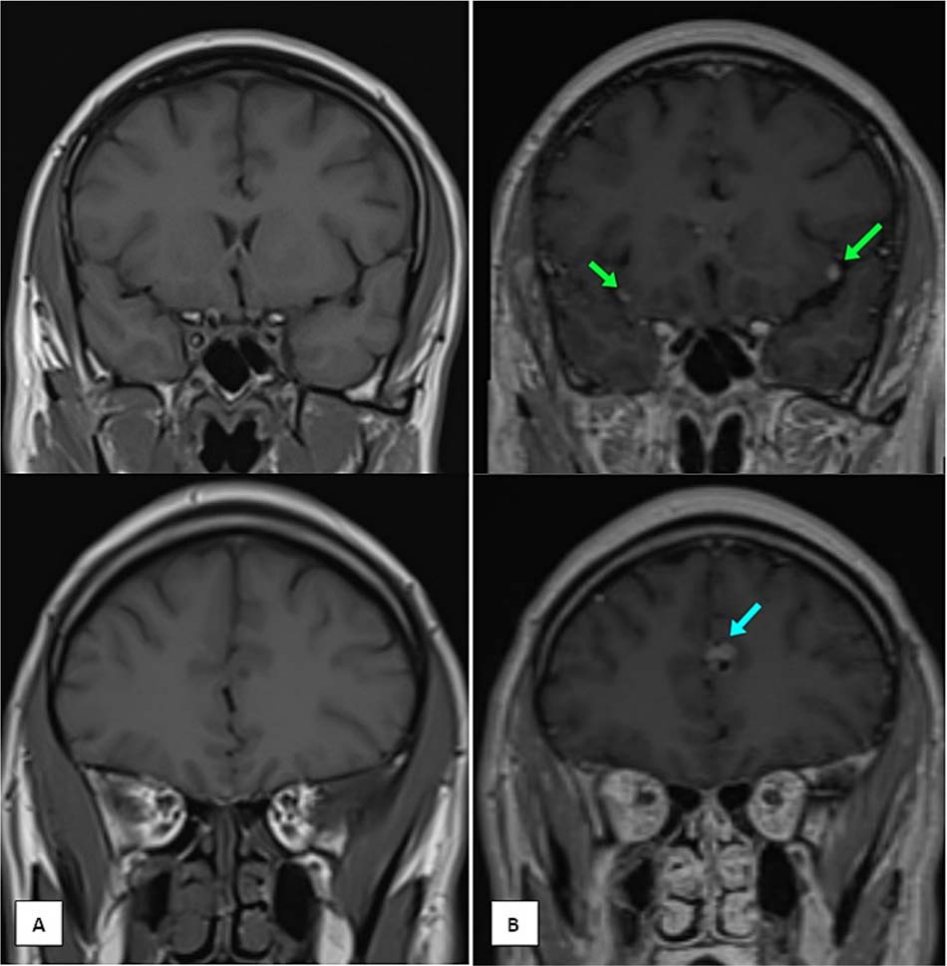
MRI of the brain (A) coronal T1 pre-contrast and (B) coronal T1 post-contrast images showing multiple leptomeningeal enhancing nodules. The largest are seen at the left frontal parafalcine region (as shown in the single arrow) and bilateral sylvian fissures (as shown in the two arrows).
